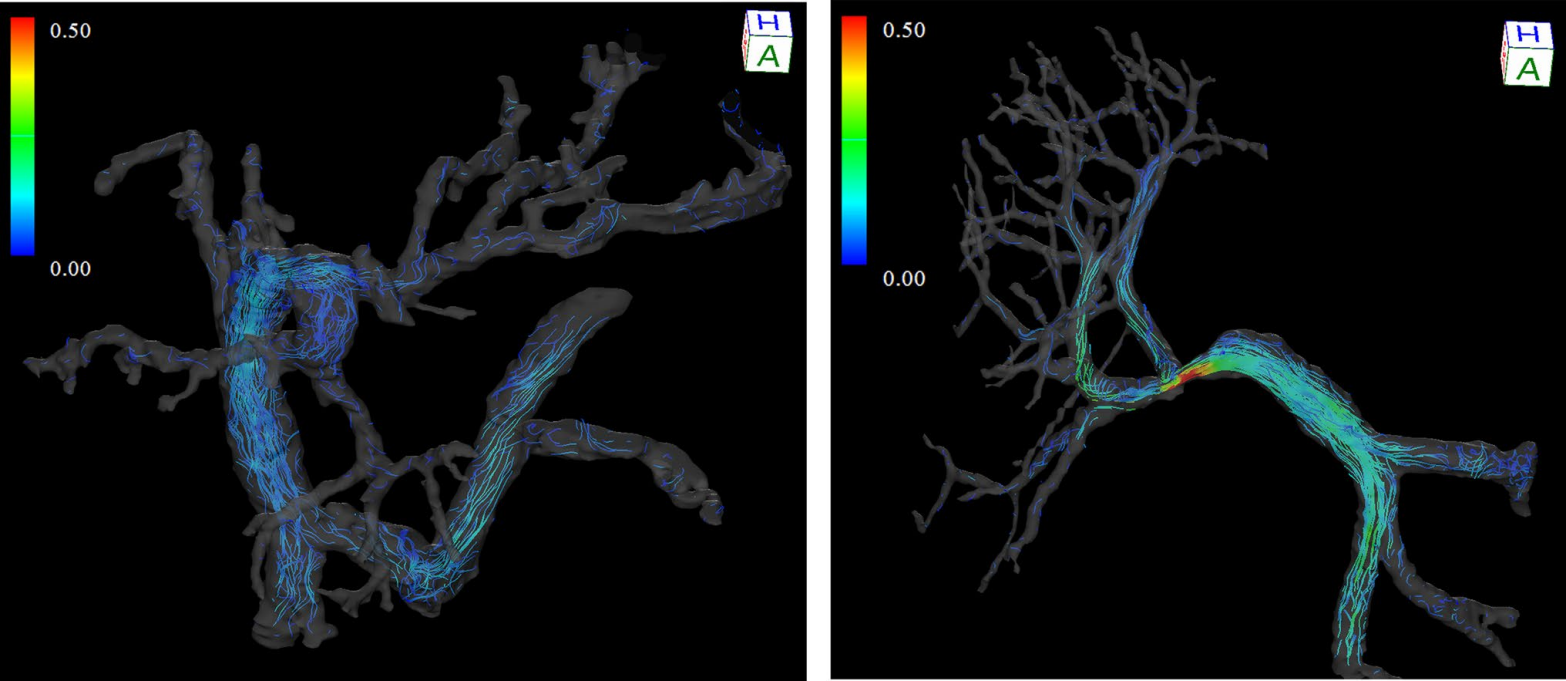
Male transplant recipient in his 50s. a The patient had severe atrophy of the right lobe of the liver and compensatory enlargement of the lateral segment of the left lobe owing to cirrhosis of unknown cause. Intrahepatic blood flow was poor except in the medial segment. To and fro blood flow was seen in the portal branches of the lateral segment. b 4D Flow MRI one week after transplantation of the recipient's liver right lobe. The right portal vein of the transplanted liver was much smaller in diameter than the recipient's main portal vein. Therefore, jet flow was observed at the anastomosis. The flow velocity in the main portal vein was increased, and a relatively homogeneous distribution of the intrahepatic blood flow was confirmed
